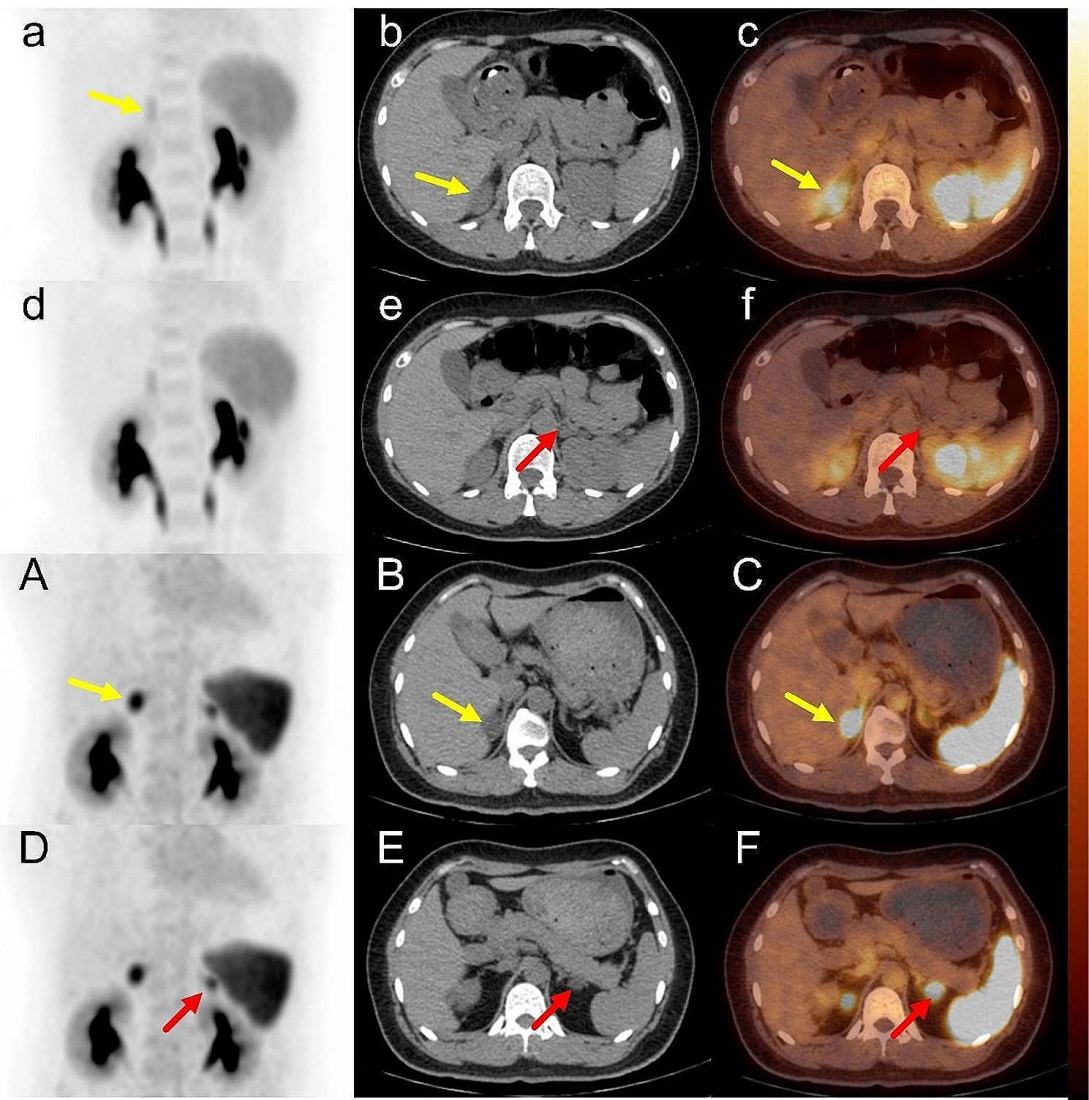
The 10-min ^68^Ga-pentixafor PET lateralization result (a-f) of patient 9 was consistent with AVS, both of which were UPA (right). a-c: right adrenal gland, d-f: left adrenal gland; right SUVmax = 5.41 (yellow arrow), left SUVmax = 4.17 (red arrow), CON = 1.30. 40 min ^68^Ga-pentixafor PET lateralization result (A-F) of patient 15 was consistent with AVS, which was BPA. A-C: right adrenal gland, D-F: left adrenal gland; right SUVmax = 12.43 (yellow arrow), left SUVmax = 11.13 (red arrow), and CON = 1.12.
